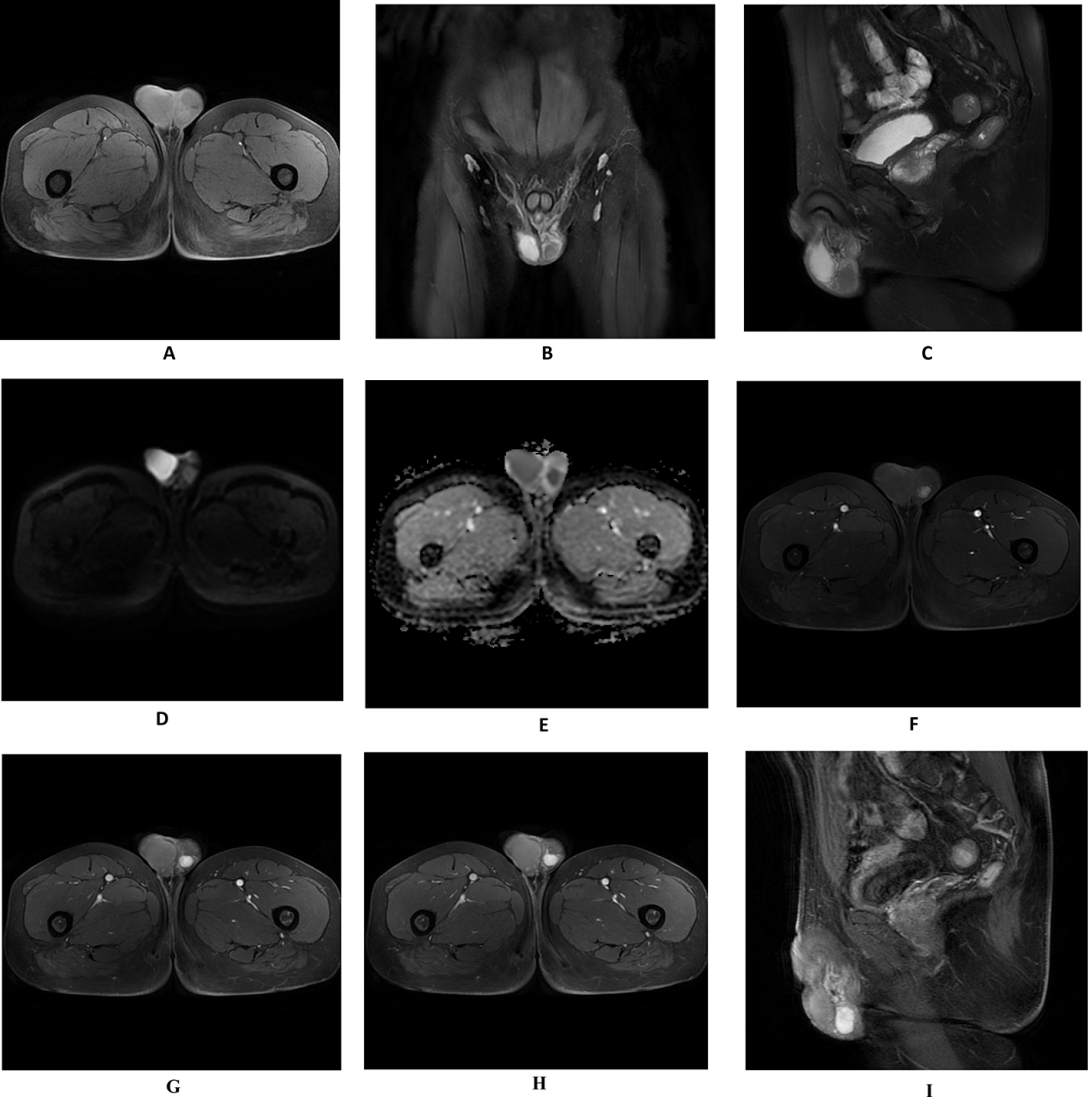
Enhanced Magnetic Resonance Imaging (MRI) scan of the pelvic cavity; Revealed a circular abnormal signal shadow in the left epididymal region, measuring approximately 16 × 12 mm, with well-defined margins. T1WI displayed iso-intense signals (A), T2WI showed iso-intense to slightly hyperintense signals (B, C). DWI exhibited high signal intensity (D), ADC map indicated low signal intensity (E). Enhanced scan demonstrated progressive enhancement (F–I)
